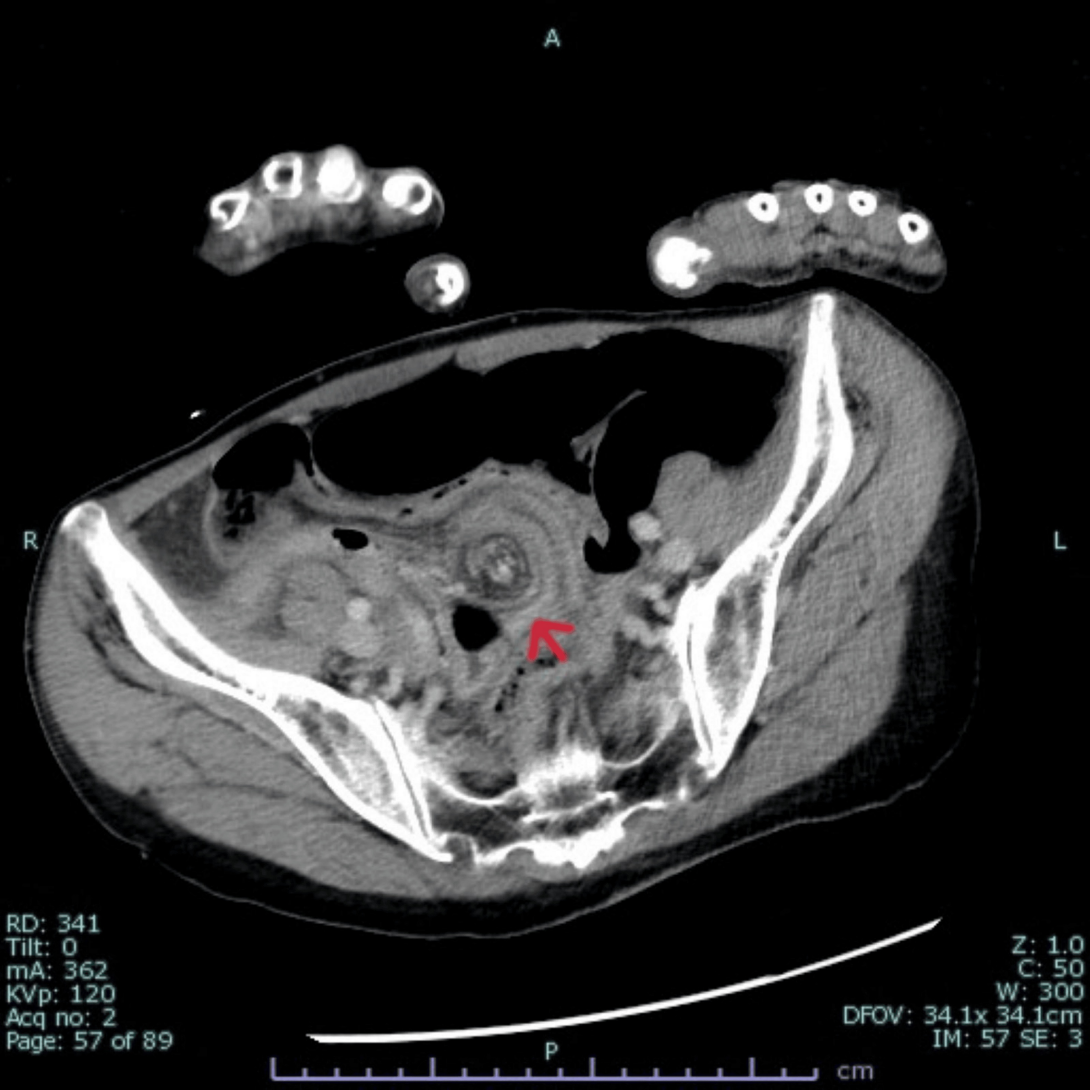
Contrast-enhanced abdominal CT on admission The arrow indicates the suspected site of mesenteric torsion.
